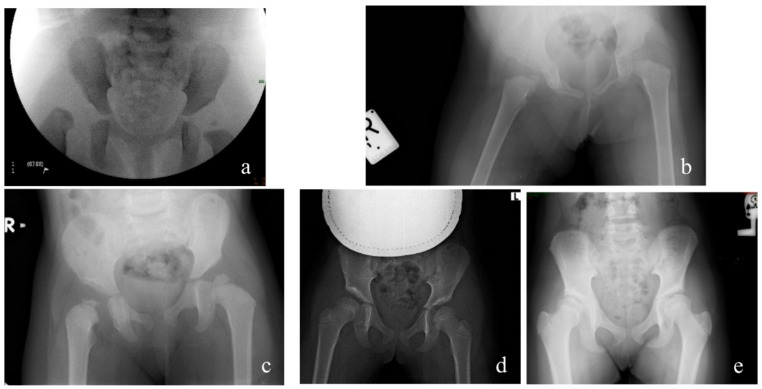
a–e Radiographic evidence of spontaneous improvement in avascular necrosis. The child underwent open reduction of the right hip at the age 1.4 years (a), At cast removal and 6 months later, signs of AVN can be seen (b, c). At 5- years follow up AVN had resolved, although the hip remained dysplastic (d). Ten years after surgery there is further improvement of dysplasia (e)
